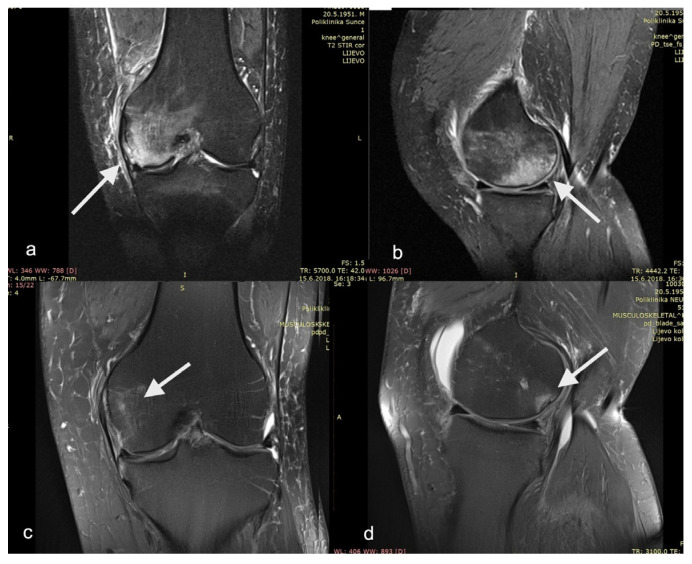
T2−weighted magnetic resonance imaging of patient’s left knee as (a) AP view and (b) LL view before surgery showing medial femoral condyle bone marrow lesion. The same patient in (c) AP view and (d) LL view at 12−month follow−up. Bone marrow lesions are show with arrows.
